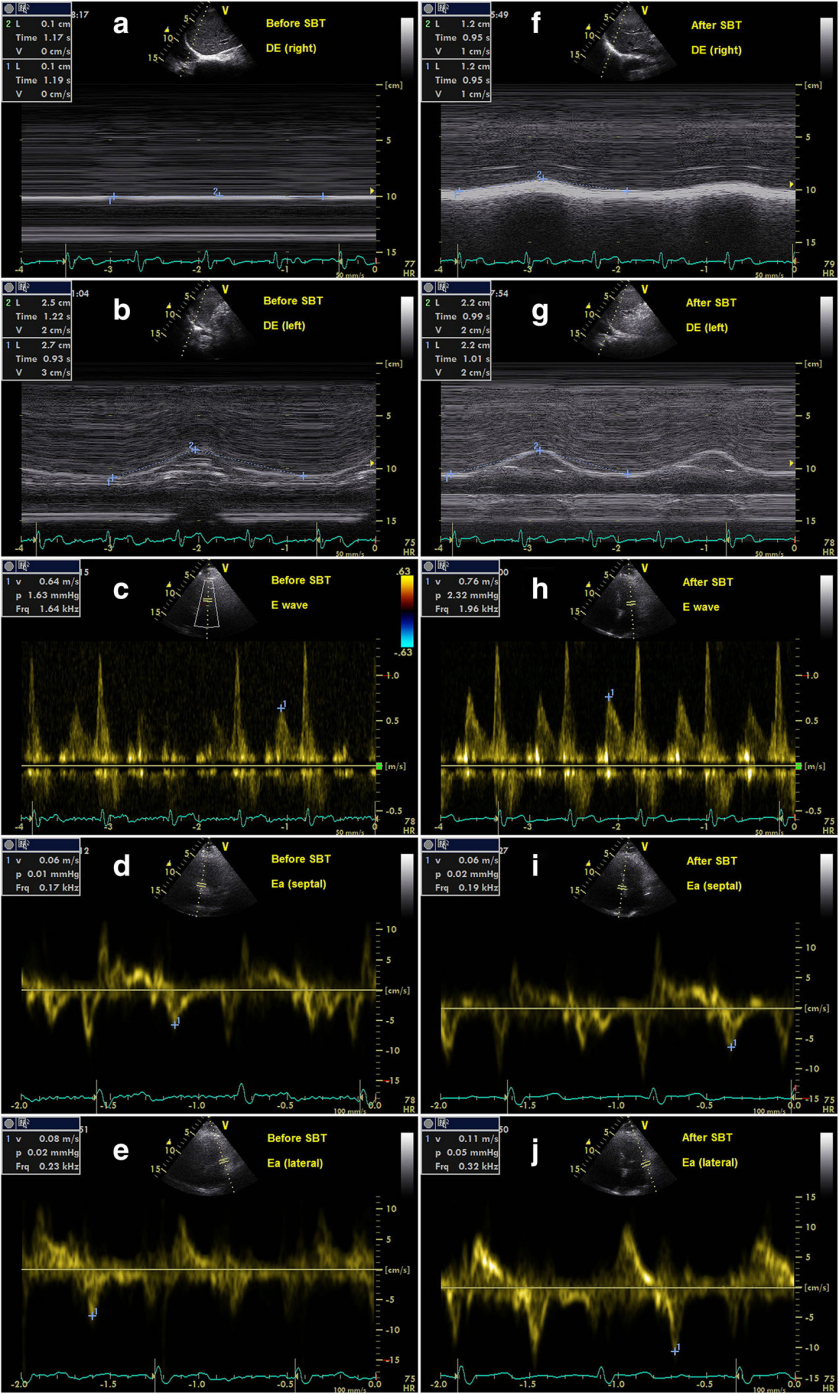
The measurements of E/Ea and DE before and after SBT in a patient of extubation success. a, b, c, d, e represented the results before SBT, and f, g, h, i, j represented the results after SBT. a DE (right) was 1 mm. b DE (left) was 26 mm. c E was 64 cm/s. d Ea (septal) was 6 cm/s. e Ea (lateral) was 8 cm/s. f DE (right) was 12 mm. g DE(left) was 22 mm. h E was 76 cm/s. i Ea (septal) was 6 cm/s. j Ea (lateral) was 11 cm/s. Before SBT, DE (average) was 13.5 mm, and E/Ea (average) was 9.1. After SBT, DE (average) was 17 mm, and E/Ea (average) was 8.9. After extubation, the patient did not develop respiratory failure within 48 h. The patient did not require re-intubation within 1 week. *DE* diaphragmatic excursion, *E/Ea* the ratio of mitral Doppler inflow velocity (E) to annular tissue Doppler wave velocity (Ea), *SBT* spontaneous breathing trial
